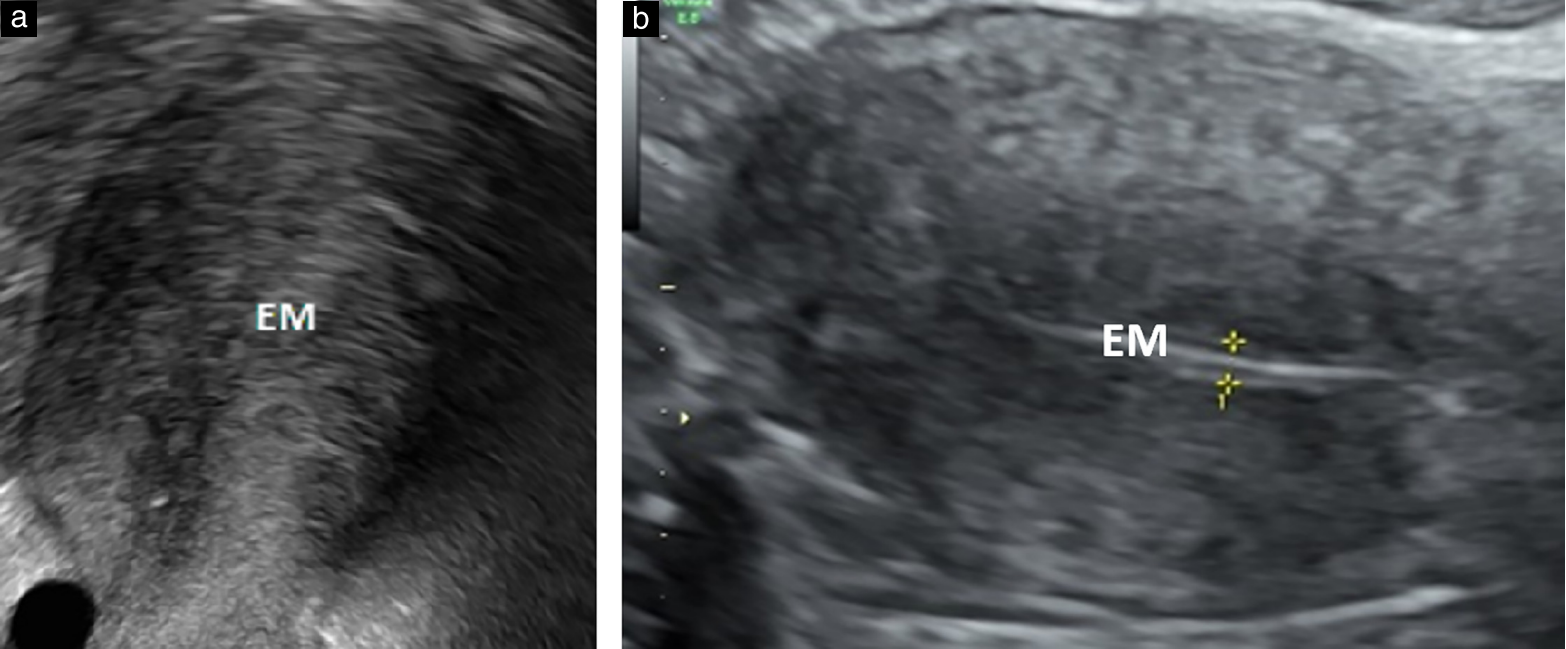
Visualization of endometrium (EM) in uterus with unfavorable position on transvaginal ultrasound (a) and on transabdominal ultrasound (multimodal approach) (b). Calipers indicate EM thickness.
